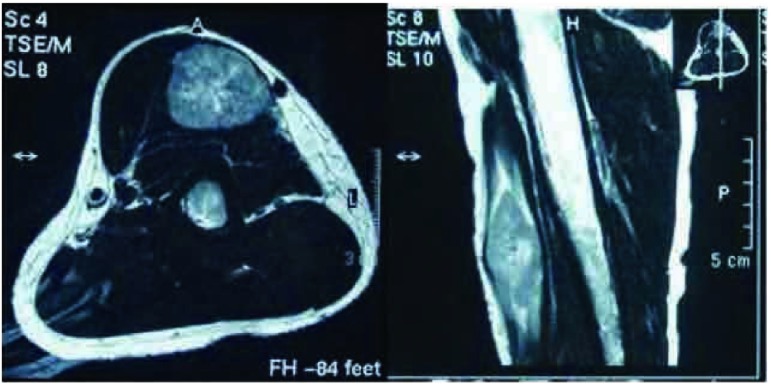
MRI检查示左上臂软组织肿物 MRI scan demonstrated a mass from soft tissue
